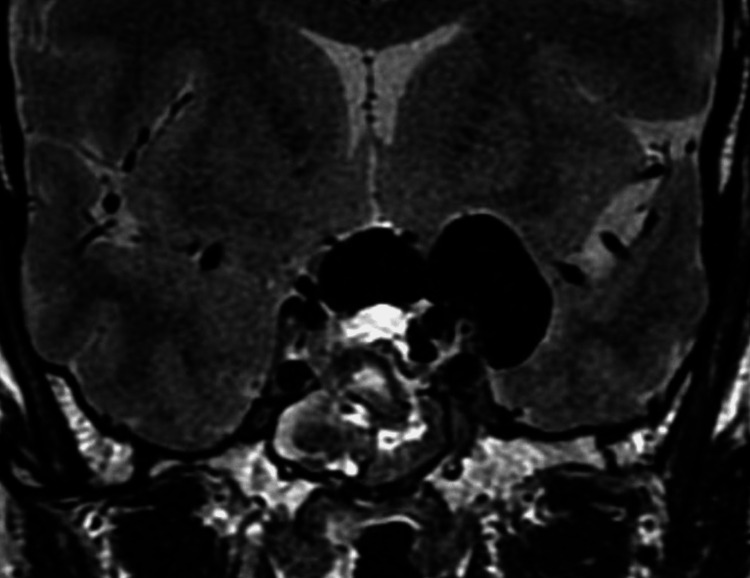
Postoperative coronal T2-weighted magnetic resonance imaging of the sellar region, showing air inside the cyst, as well as a significant reduction of the size of the cyst
